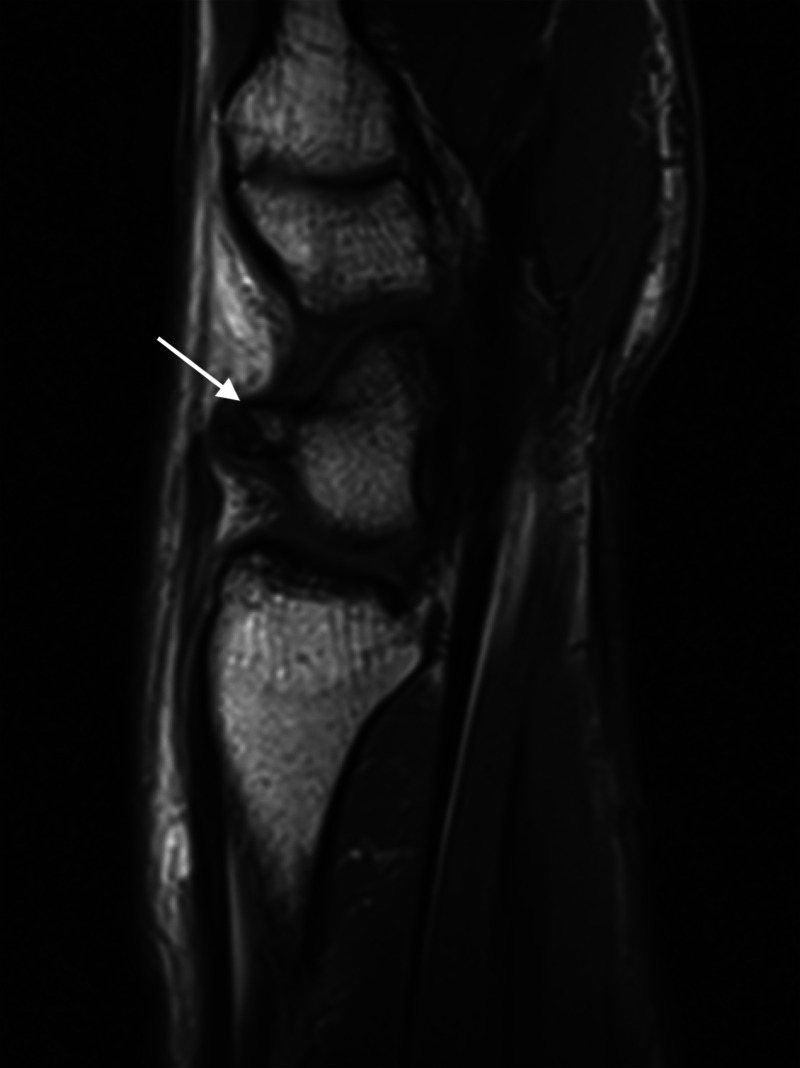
Sagittal Magnetic Resonance Image of the Radial Wrist. An avulsive cortical irregularity of the dorsal scaphoid at the extrinsic ligamentous insertion, likely of the dorsal intercarpal ligament (white arrow). The bones demonstrated no marrow signal abnormality, joint spaces were preserved, and the scapholunate ligament was intact.
